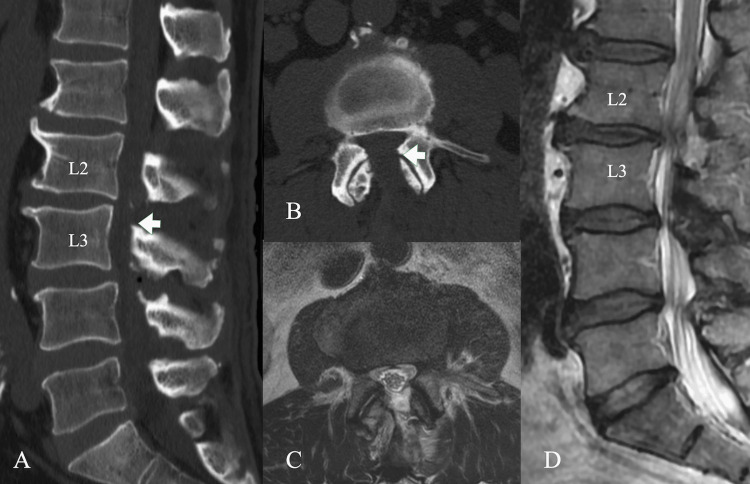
Postoperative imaging (A) Sagittal CT confirmed that the osteophytes were resected (arrow). (B) Axial CT confirmed the osteophytes extending from the superior articular process were resected (arrow), with bony resection on both the entry and contralateral sides. (C) Axial MRI showed that the ligamentum flavum was removed on both sides. (D) Sagittal MRI showed decompression at the L2 and L3 levels.
